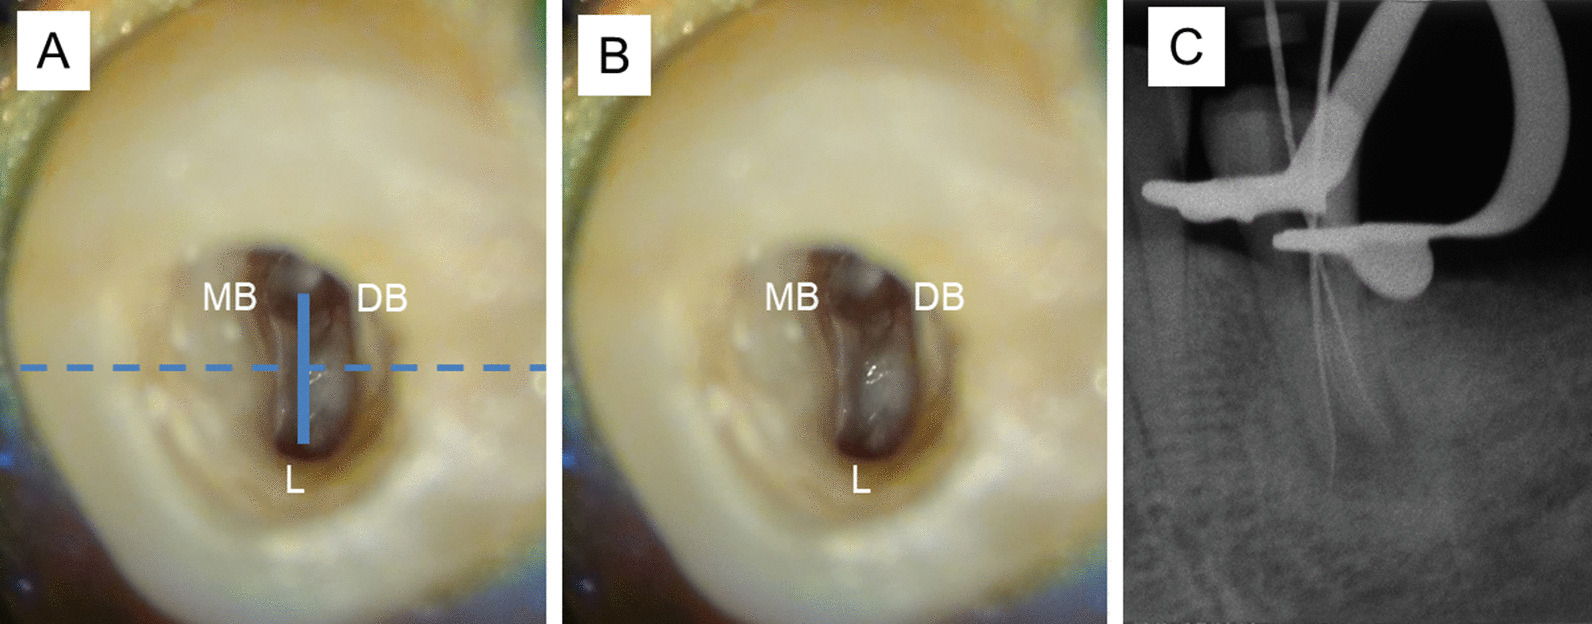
Five Krasner and Rankow laws can be applied in trifurcated left mandibular first premolars, A Law of symmetry 1: the mesiobuccal (MB) and distobuccal (DB) root canal orifices are equidistant to the lingual (L) orifice on a line draw in a mesial-distal direction across the center of the floor of the pulp chamber (M-D line), Law of symmetry 2: the orifices of the canals lie on a line perpendicular to M-D line. B Law of Color Change: the color of the pulp-chamber floor is always darker than the walls, Law of Orifice Location 1 and 2: the orifices of the root canals are located at the angles in the floor-wall junction, and Law of Orifice Location 3: the orifices of the root canals are located at the terminus of the root developmental fusion lines, C a radiograph with files inside the root canals from left to right are mesiobuccal (MB), distobuccal (DB), and lingual (L) root canal respectively. Dashed line indicates M-D line and the solid line indicates the line perpendicular to M-D line
